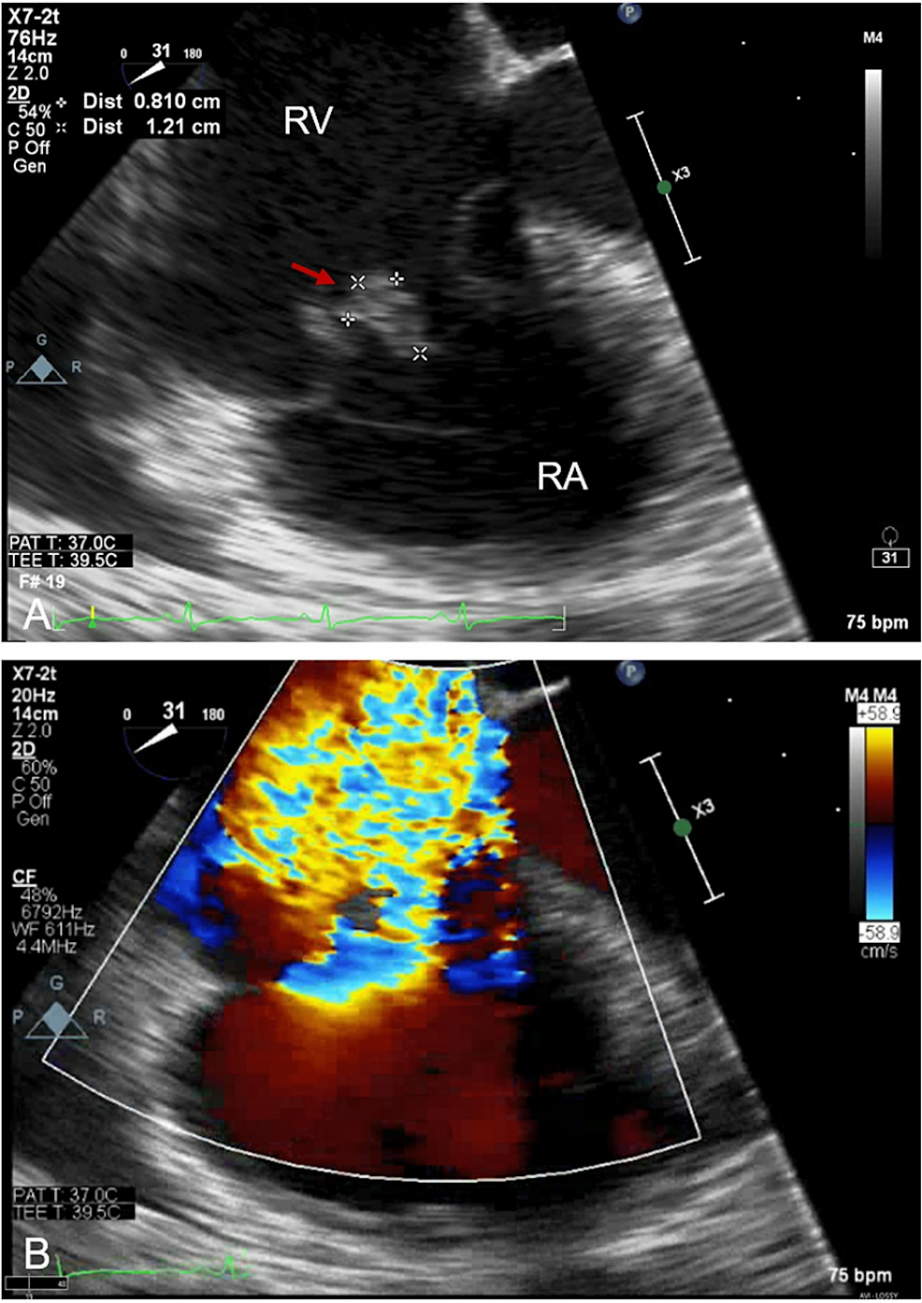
(A) Preoperative TEE demonstrating a vegetation on the anterior leaflet of the tricuspid valve (arrow). (B) Preoperative TEE with color Doppler showing a flail anterior leaflet of the tricuspid valve with severe tricuspid regurgitation. RV: right ventricle; RA: right atrium; TEE: transesophageal echocardiogram.
